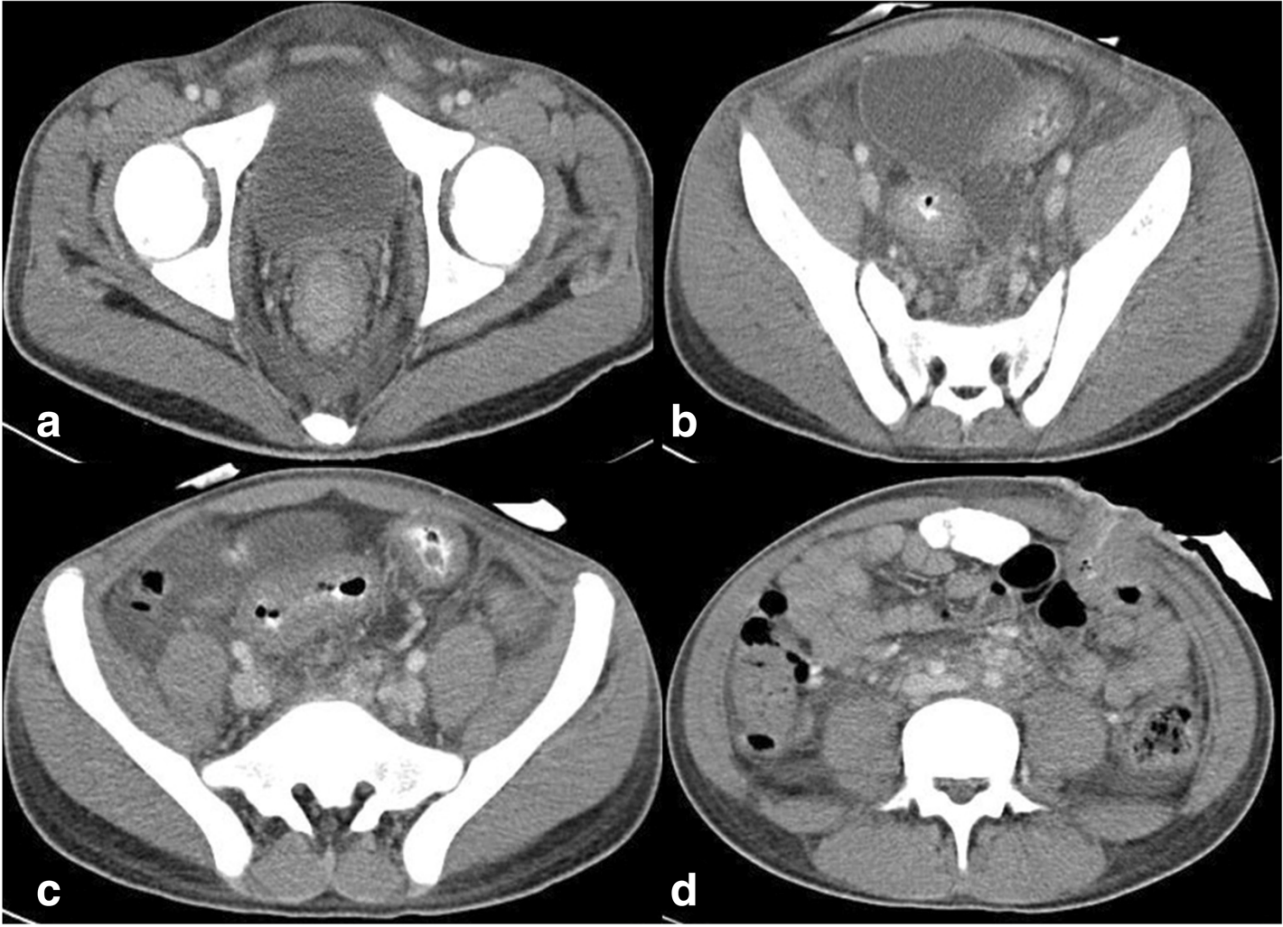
Contrast-enhanced computed tomography of the abdomen and pelvis. a–c Circumferential growth involving rectum and sigmoid colon. d Colostomy site
